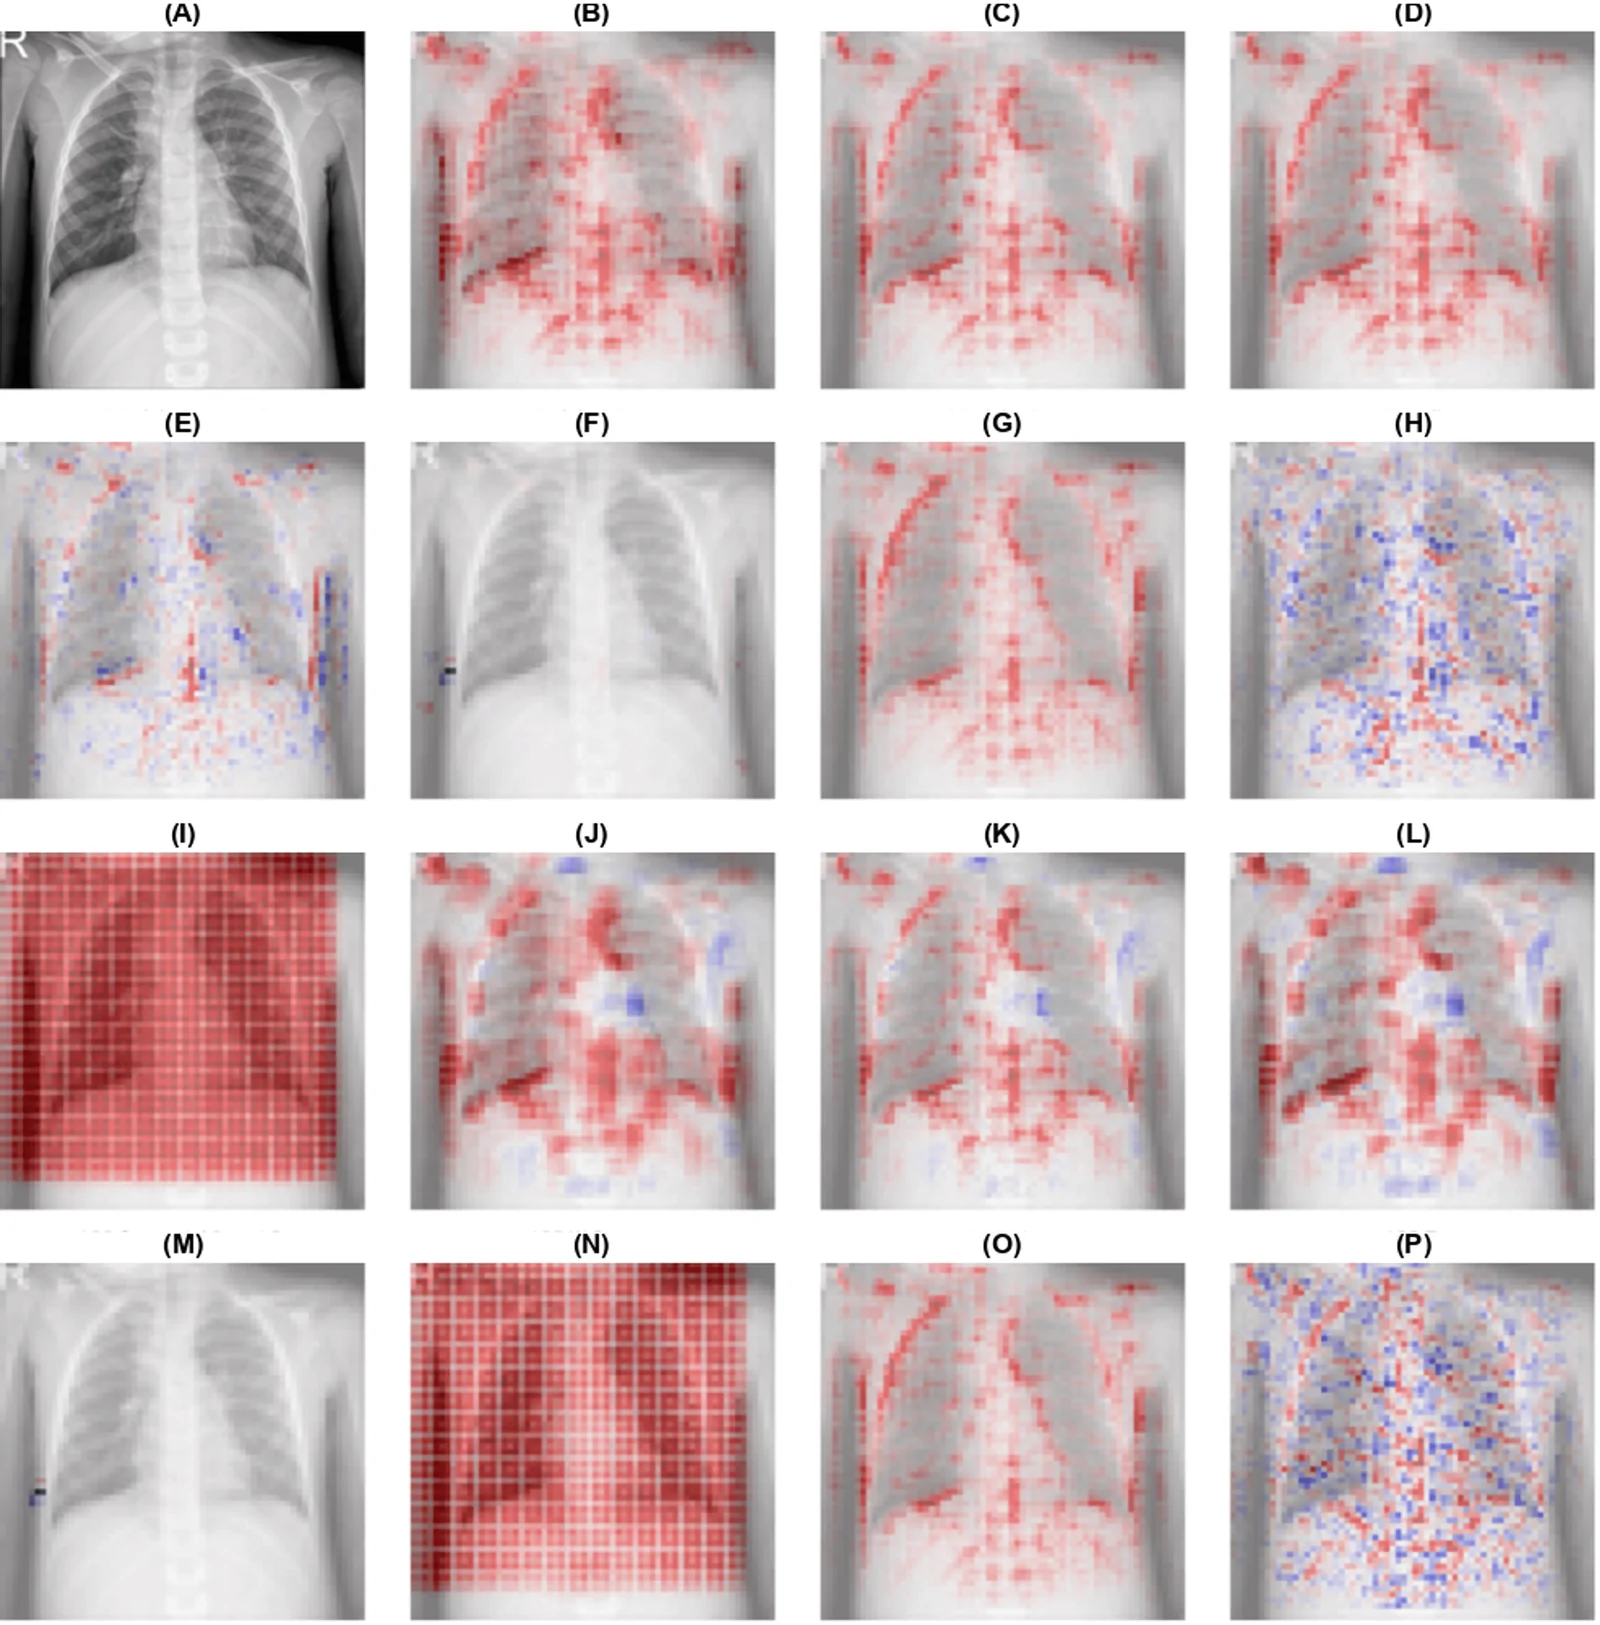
Representative pneumonia chest X-ray analyzed with Deep Taylor and LRP rules for the 3-class model (healthy, pneumonia, and COVID-19). Panels show the original image and corresponding relevance maps generated by each explanation method. (A) Original chest X-ray; (B) Deep Taylor Bounded; (C) Deep Taylor; (D) LRP Alpha 1 Beta 0; (E) LRP Alpha 2 Beta 1 IB; (F) LRP Alpha 2 Beta 1; (G) LRP Alpha Beta; (H) LRP Epsilon; (I) LRP Flat; (J) LRP Sequential Preset A Flat; (K) LRP Sequential Preset A; (L) LRP Sequential Preset B Flat; (M) LRP Sequential Preset B; (N) LRP W Square; (O) LRP Z Plus; (P) LRP Z. LRP: layer-wise relevance propagation; IB: input-layer bounded. Panel (A) was adapted from a case contributed by Yaïr Glick, Radiopaedia.org, rID 92316 [20], and is used under the Creative Commons Attribution-NonCommercial-ShareAlike 3.0 Unported license (CC BY-NC-SA 3.0); all relevance maps and annotations were generated by the authors.
